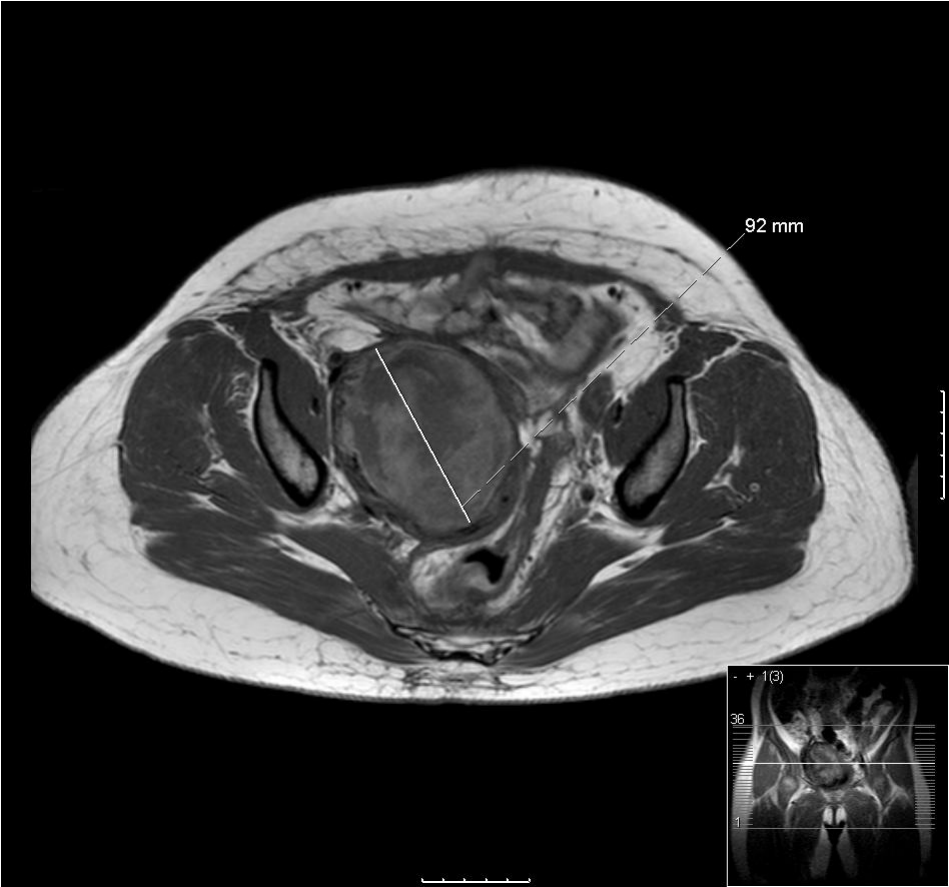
MRI scan of the pelvis prior to starting sirolimus, following total abdominal hysterectomy and bilateral salpingo-oophorectomy (TAH BSO), demonstrating the perivascular epithelioid cell tumor (PEComa) measuring 92 mm.
